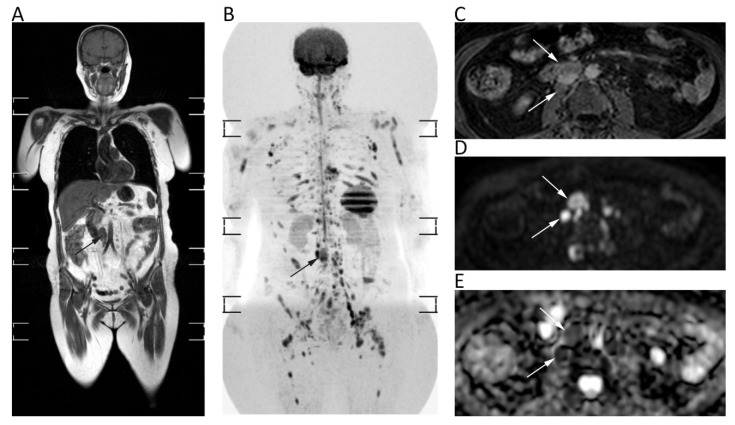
Whole-body MRI of a 58-year-old female patient with non-Hodgkin lymphoma. Whole-body T1w (A) and coronal MIP grey-scale inverted DWI (B) show multiple nodal and bone locations of disease. Note the periaortic lymph nodal locations (arrows) with moderate contrast enhancement on axial fat-suppressed 3D GRE T1w (C) and restricted pattern of diffusion on axial *b* = 800 DWI (D) and corresponding ADC map (E, mean ADC: 0.761 × 10^−3^ mm^2^/s).
